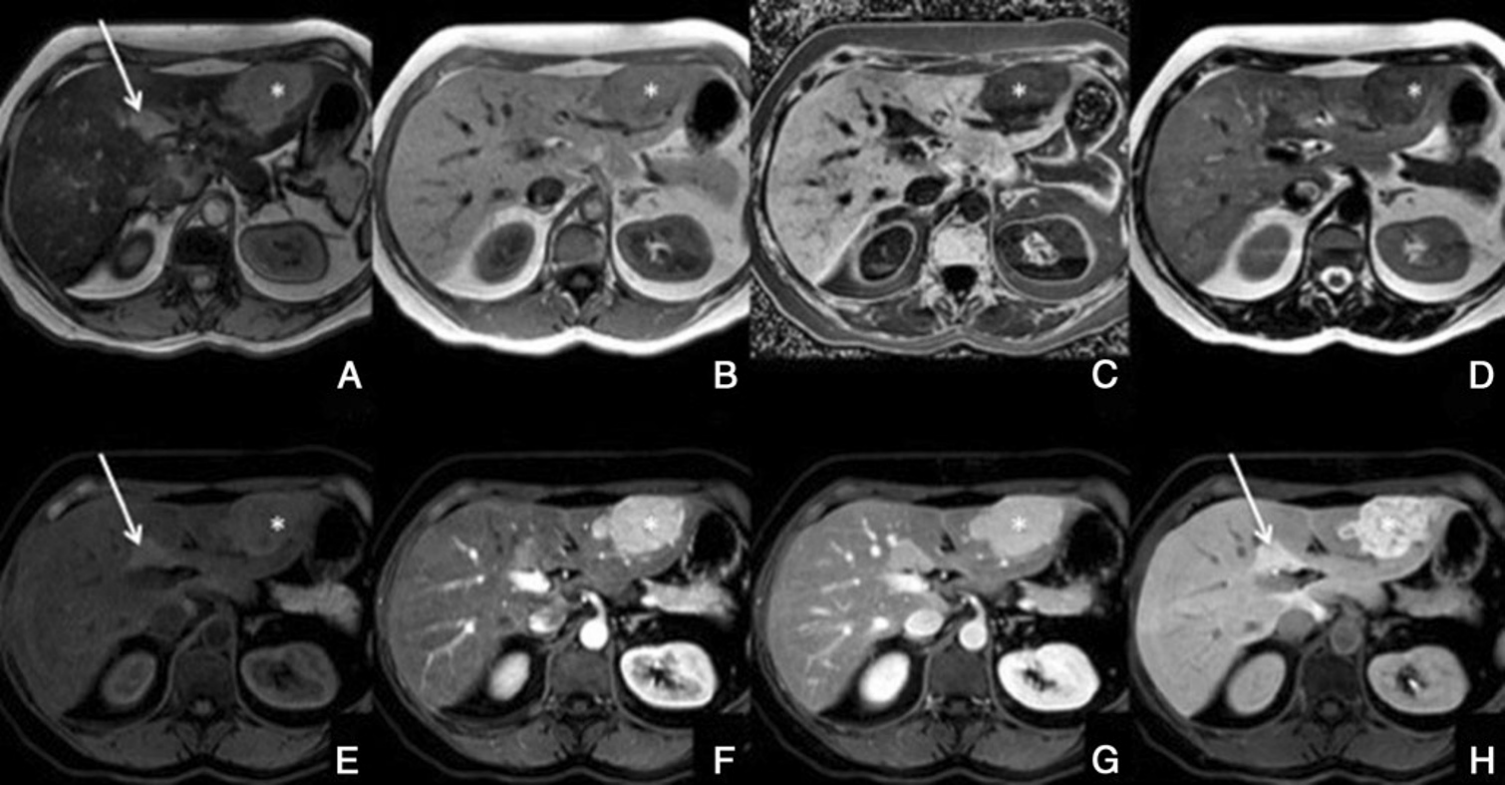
Focal fat sparing area and a FNH, in a 36-year-old woman with no liver disease. a OP, b IP, c PDFF, d T2-weighted, e T1 fat-sat, f arterial phase, g PV phase, h HCP. Triangular-shaped periportal area (*arrows*) hyperintense to the rest of the liver in OP and Fat Sat imaging mimicking uptake of Gd-EOB-DTPA, corresponding to a fat spared area. This is confirmed in the fat-fraction map. Of note, is a lesion (*) compatible with a FNH
